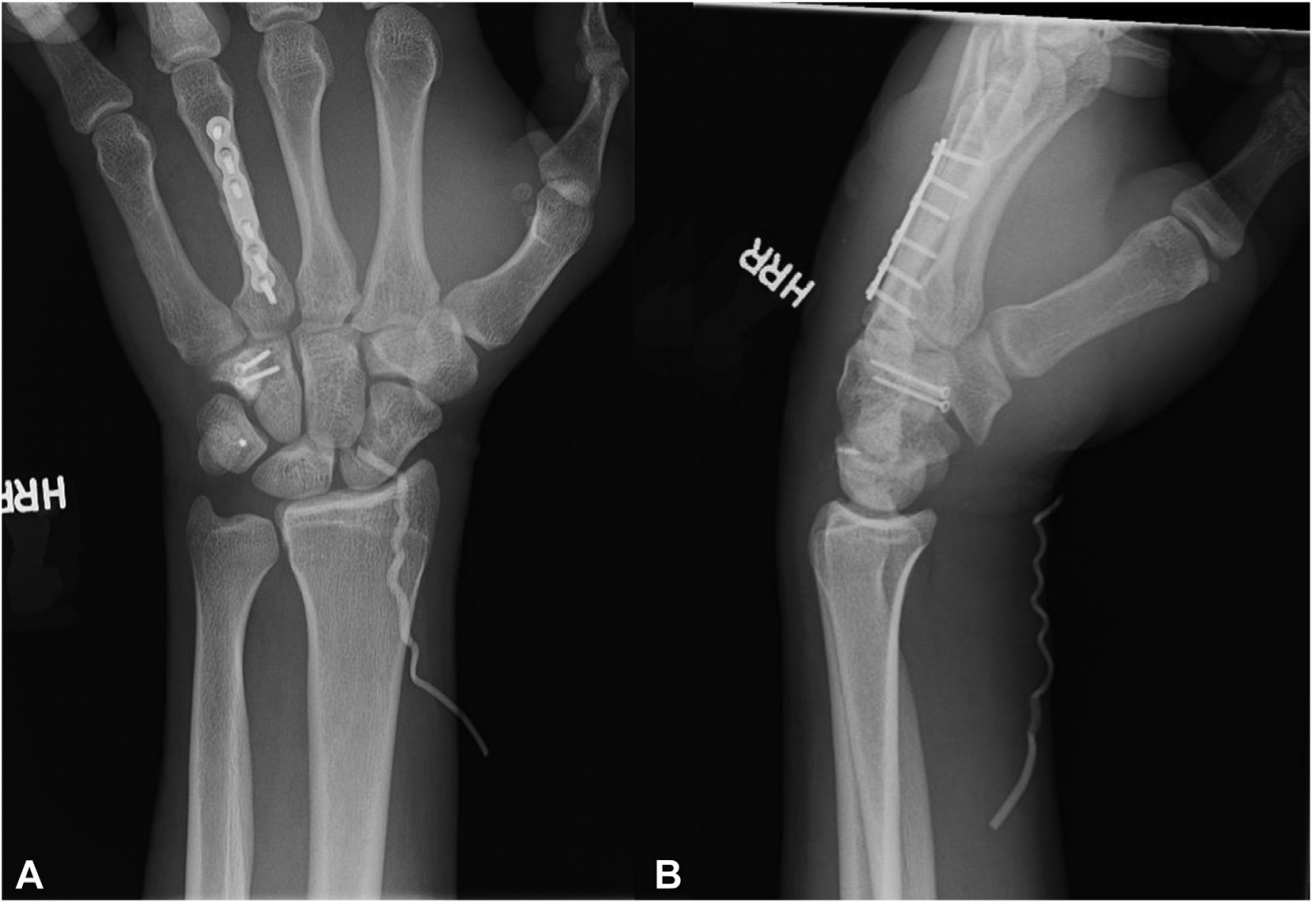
Two radiographic views of the left wrist. A Posterior-anterior view. B Lateral view. These images demonstrate optimal reduction and fixation of the patient’s multiple injuries. A vessel loop used to aid in superficial suture removal can be visualized.
